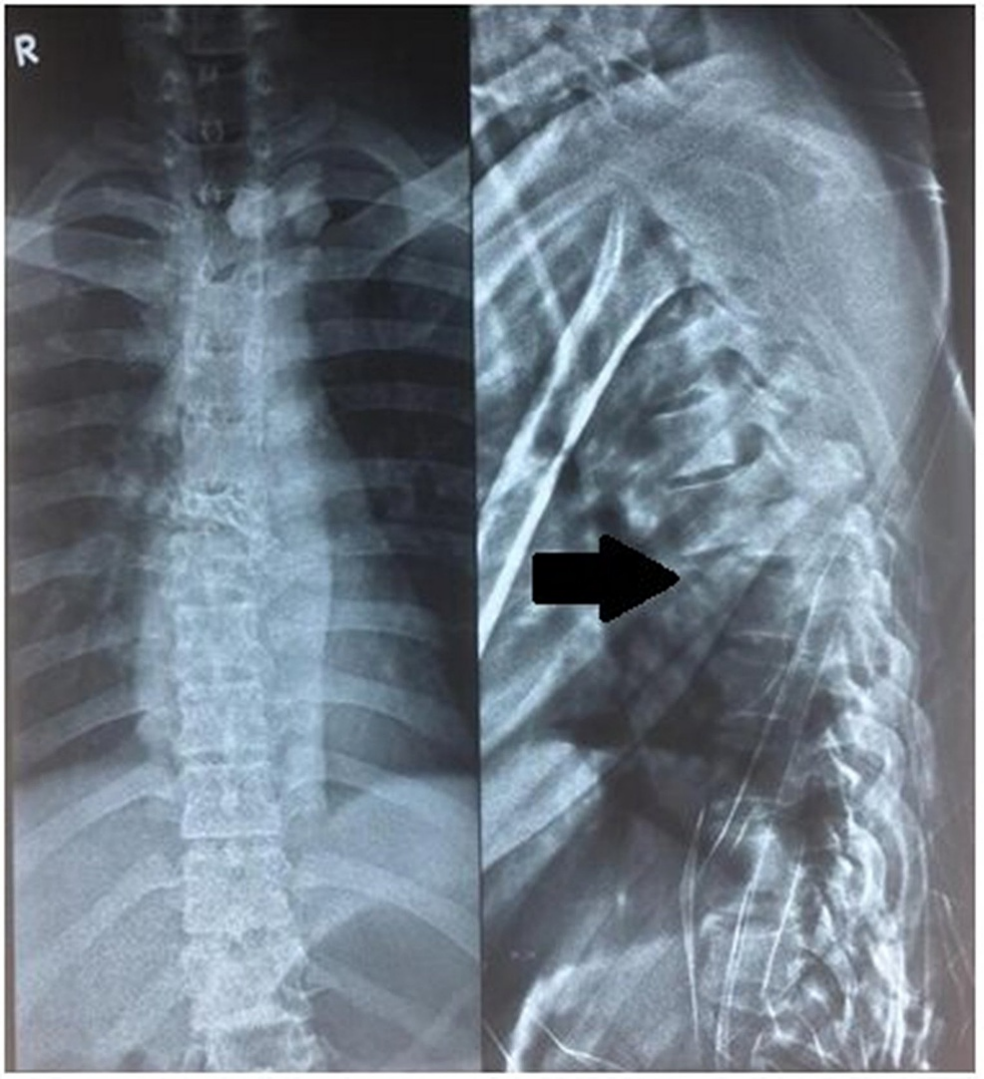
Preoperative radiograph (AP and lateral) of thoracic spine showing T6-T7 fracture-dislocation (black arrow) AP - anteroposterior
